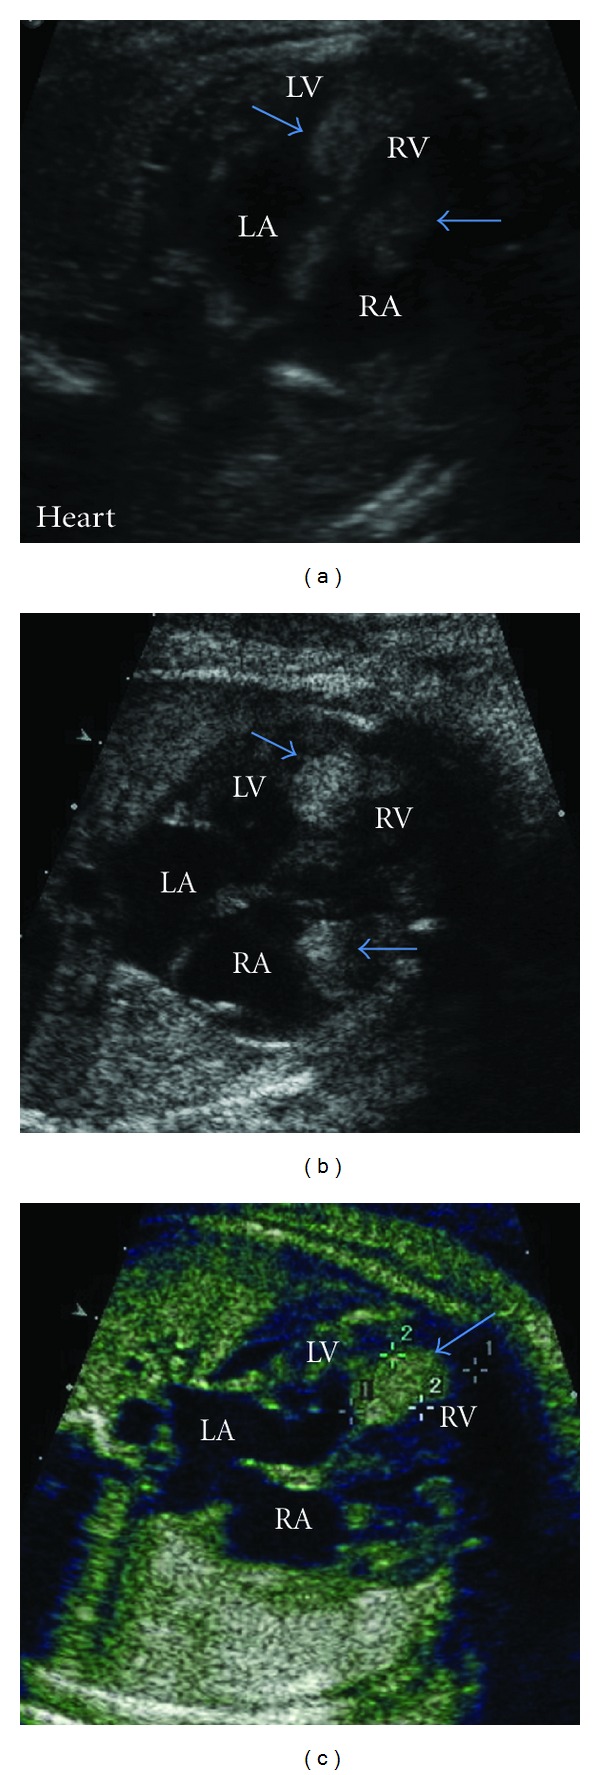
Multiple intracardiac masses (arrows) were detected on routine ultrasound (a), prompting a more thorough investigation. Fetal echocardiography ((b) and (c)) identified five masses that were within the ventricular myocardium although only two (arrows) can be seen in this plane. The masses were of uniform echogenicity and were more echogenic than the surrounding myocardium, consistent with the typical appearance of cardiac rhabdomyomas.
